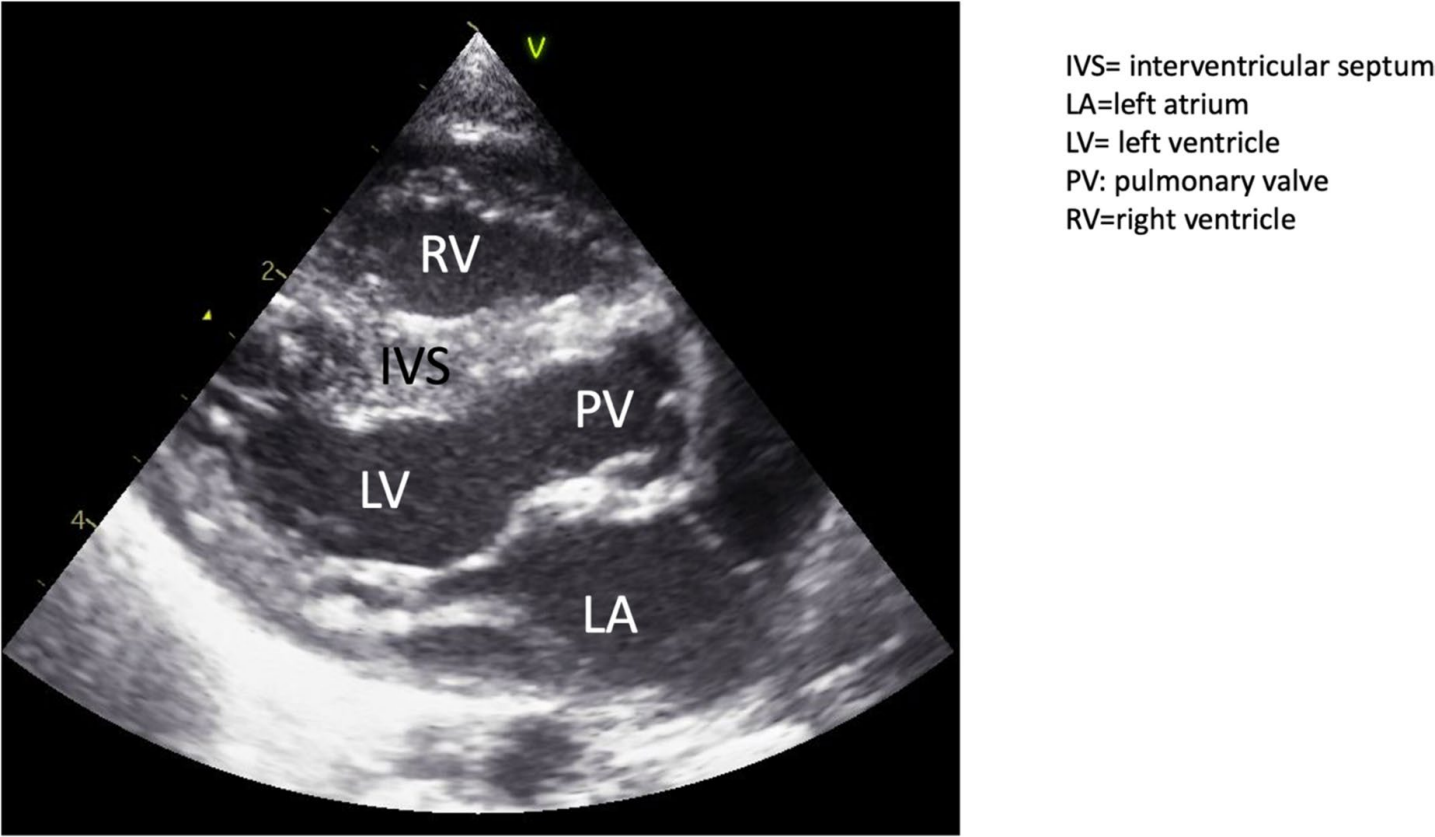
Echocardiographic long-axis view with no VSD visible
